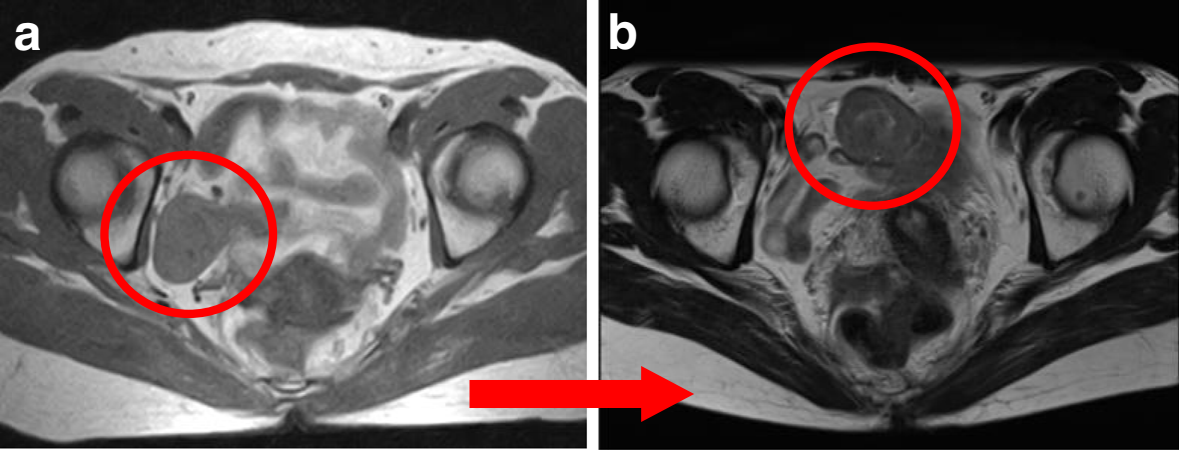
Preoperative pelvic T1-weighted MRI findings. A tumor mass of 37 mm in size was found in the pelvis, and a mobile lesion in the peritoneal cavity was observed by comparing the image taken at the referral hospital (a) with the image taken at our hospital (b). The tumor appeared at a mildly higher signal than the muscle
